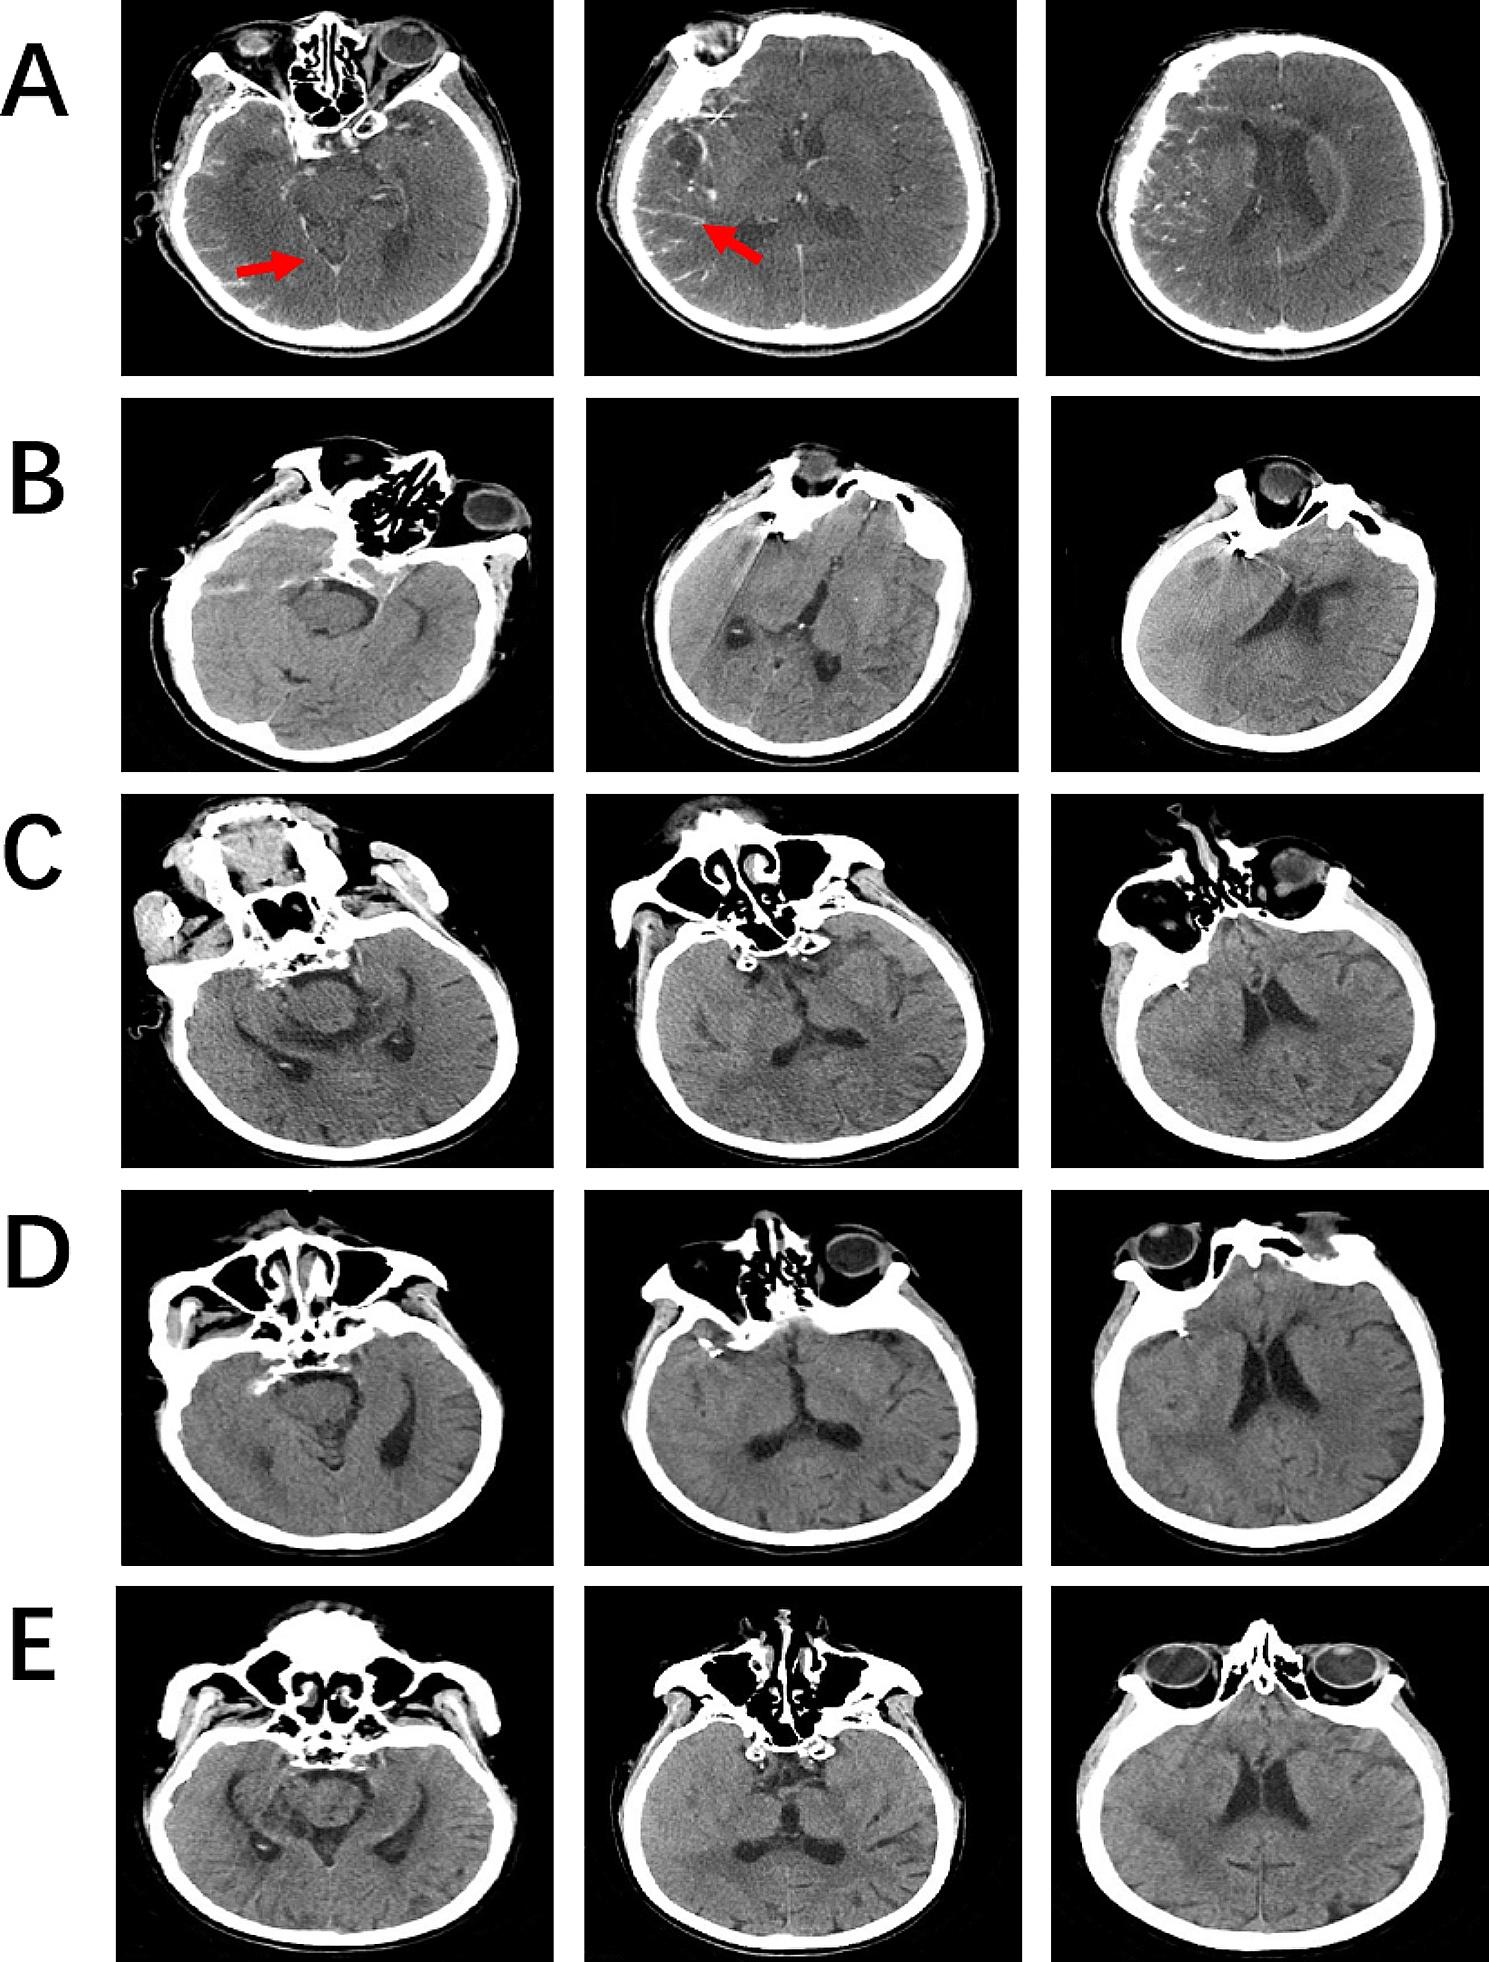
Brain CT scans of the CIE patient. (A) The Xper-CT scan was performed immediately post-operation. (B) The brain CT scan was performed after 7 h of the procedure. (C) The brain CT scan was performed the following day post-procedure. (D) The brain CT scan was performed on the 4th day post-procedure. (E) The brain CT scan was performed on the 6th day post-procedure
